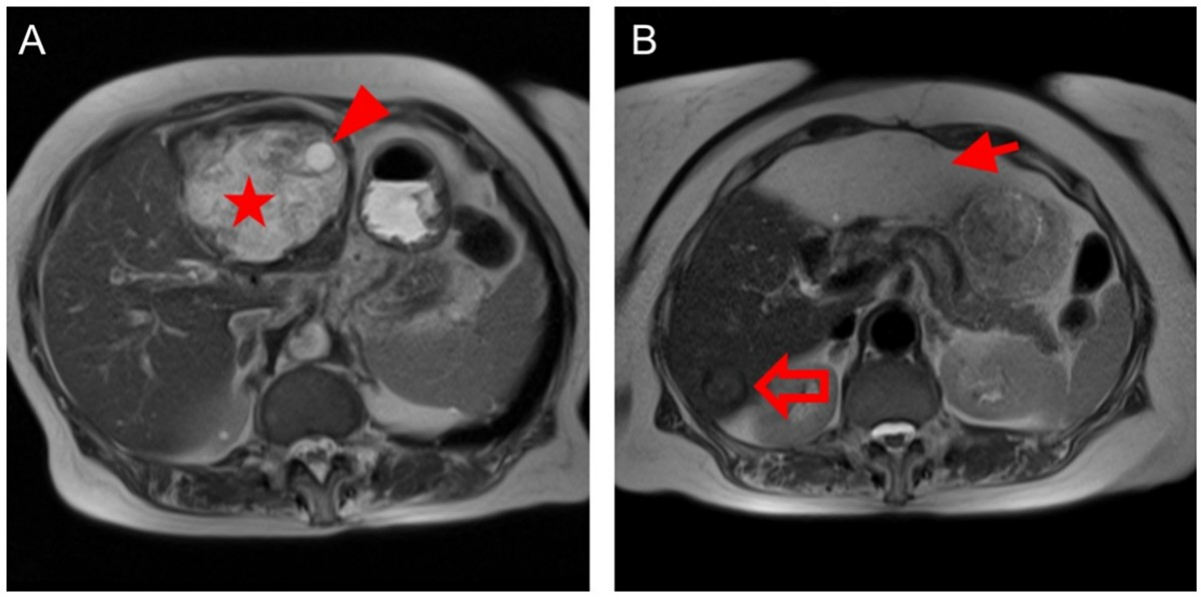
Patient with hepatic cystic echinococossis WHO stage CE3b. A: The preoperative T2-weighted MRI scan shows a large cystic mass in the left liver lobe that has a heterogeneous content including solid components (star symbol) and one daughter vesicle (arrow head) corresponding to a WHO CE3b cyst. B: The postoperative T2-weighted MRI scan shows the omentoplasty within the residual endocystectomy cavity (arrow). The open arrow points to an inactive WHO CE5 cyst in the right liver lobe.
